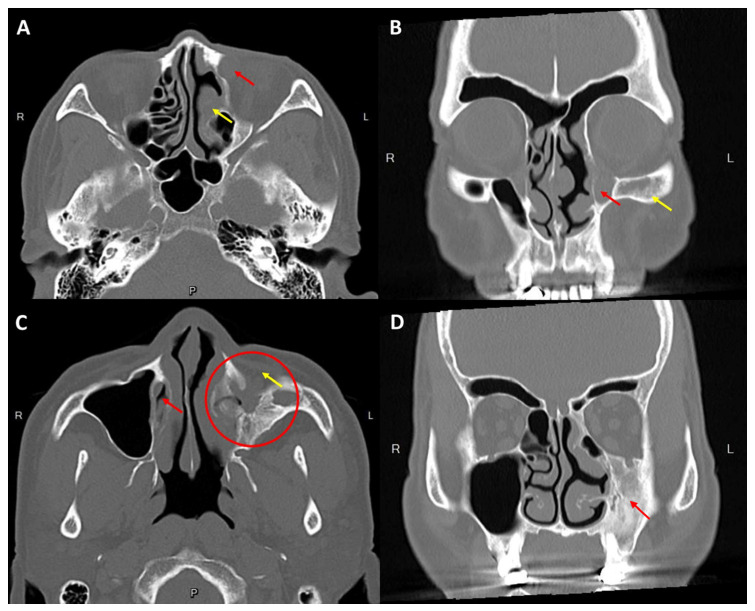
(A) Axial section through the lacrimal sac shows an expanded lacrimal sac (red arrow) and ethmoidectomy with superior turbinate hypertrophy on the left (yellow arrow). (B) Coronal computed tomography (CT) scan shows a prominent dilated intraosseous part of the left nasolacrimal fluid-filled duct (red arrow) and osseous proliferation of the left maxillary sinus (yellow arrow). (C) The lower axial section shows the intact terminus of the nasolacrimal canal on the right, within the inferior nasal meatus (red arrow), and totally obliterated on the left due to osseous proliferation and shrinkage of the left maxillary sinus (red circle). A bony defect of the anterior sinus wall (yellow arrow) was also observed. (D) The coronal CT scan shows osseous thickening, sinus retraction, and cavity volume reduction on the left maxillary sinus (red arrow) and increased volume of the left orbit.
